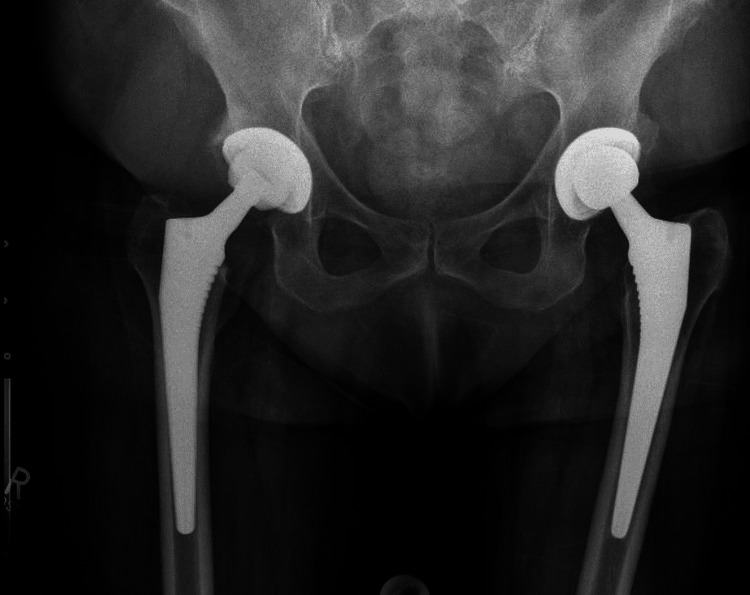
Postoperative anteroposterior radiograph demonstrating satisfactory component positioning and alignment after implantation of the right total hip arthroplasty.
